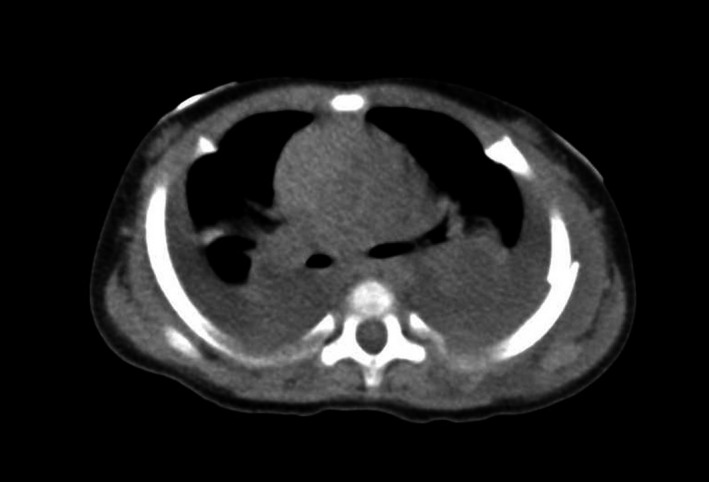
Chest computed tomography shows bilateral pleural fluid and rib fracture.
